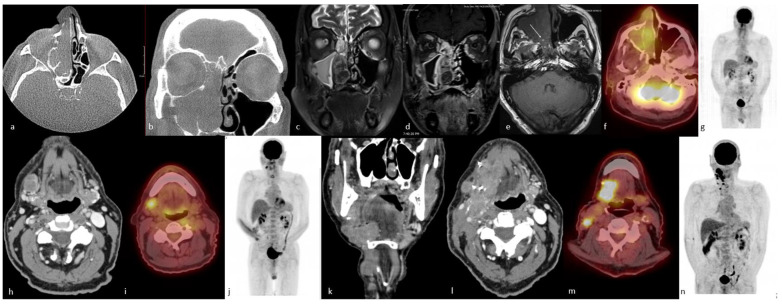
Nasal cavity malignant melanoma. Initial non-contrast CT images (a,b) show an expansile, destructive soft tissue lesion in the right nasal cavity, resulting in destruction of the right turbinates and ethmoid septae. The right medial maxillary sinus is dehiscent, with opacification of the right maxillary sinus. The cribriform plate is intact. Coronal fat-saturated T2w and post-contrast T1w images (c,d) show an enhancing mass primarily in the right nasal cavity without nodular dural enhancement to suggest intracranial extension. The axial non-contrast T1w image (e) shows focal areas of hyperintensity (arrow), which may represent mucinous components, melanin, or hemorrhagic content. Subsequent ^18^F-FDG PET/CT ((f)—axial fused, (g)—MIP) shows FDG avidity of the primary lesion without distant metastasis. A biopsy revealed malignant melanoma. The patient underwent endoscopic resection, medial maxillectomy, and total ethmoidectomy and completed radiotherapy. Follow-up CT (h) and ^18^F-FDG PET/CT ((i)—axial fused, (j)—MIP) show new necrotic right submandibular lymphadenopathy without sign of local recurrence at the right nasal cavity. The patient underwent a subsequent selective right neck dissection. Follow-up CT (k,l) and ^18^F-FDG PET/CT ((m)—axial fused, (n)—MIP) 6 months after neck dissection revealed lymphadenopathy in the right neck, including conglomerate lymphadenopathy in the submental, submandibular, and sublingual spaces and the root of the tongue.
